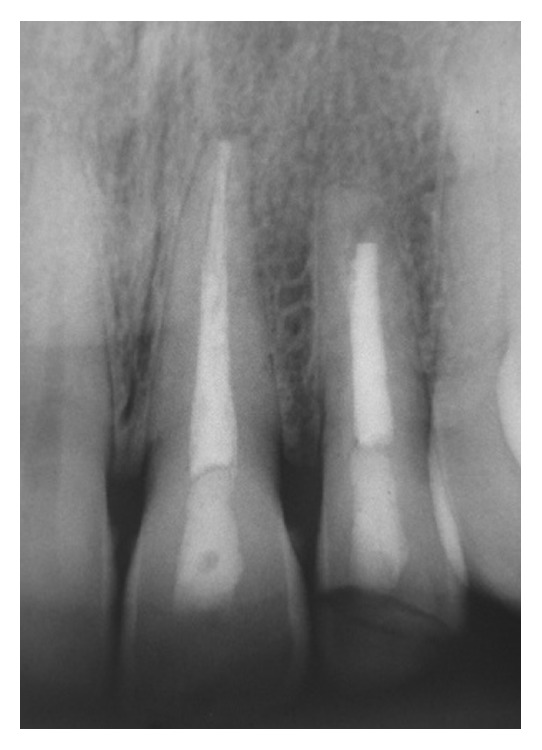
Radiograph showing the long-term success of apexification and root canal treatment of tooth 22 after fourteen and half years of follow-up and root canal treatment of tooth 21 after fifteen and half years (October 2013).
